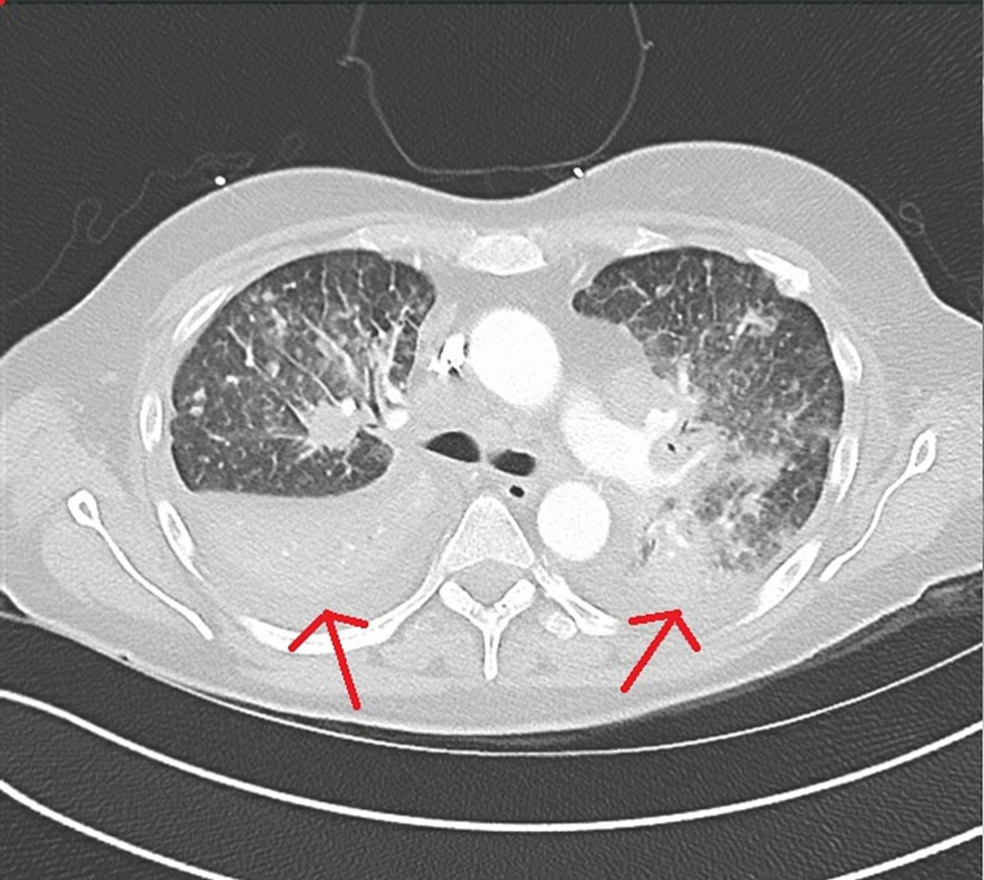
CT angiogram of the chest No evidence of acute pulmonary embolism. Innumerable pulmonary nodules with underlying lymphangitic carcinomatosis in the bilateral lungs. Bilateral mediastinal and hilar lymphadenopathy. Likely represent metastatic disease. Arrows showing moderate bilateral pleural effusions persist with atelectasis/consolidation of the right lower lobe, minimally increased since the prior examination.
